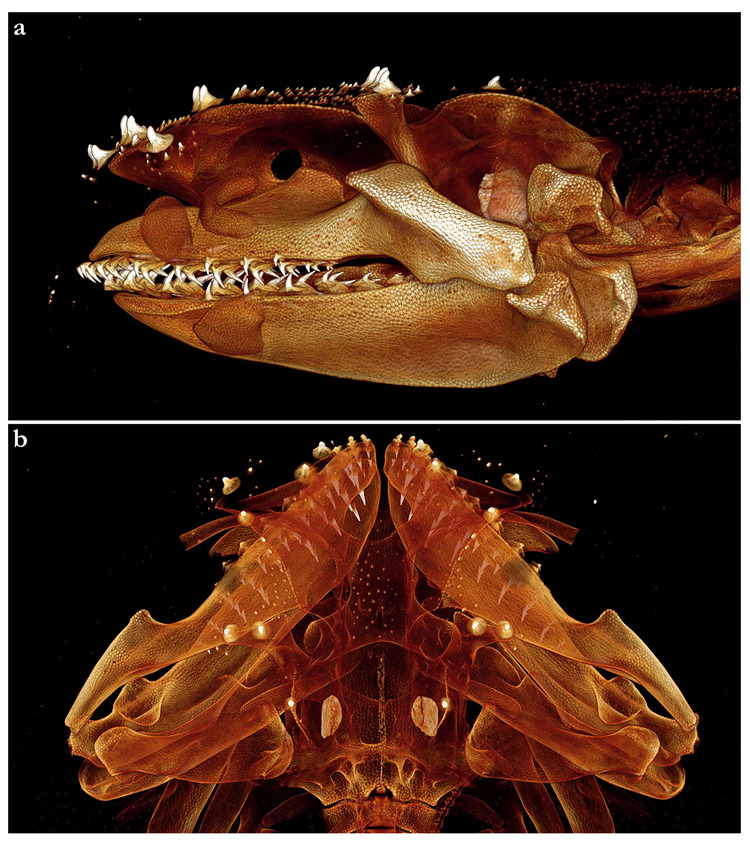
Squatina leae* sp. nov., paratype, ZMH 26098, juvenile male, 249.6 mm TL; micro-computed tomography scans of the skull in (a) lateral and (b) dorsal views. Anterior to left in (a) and top in (b).
